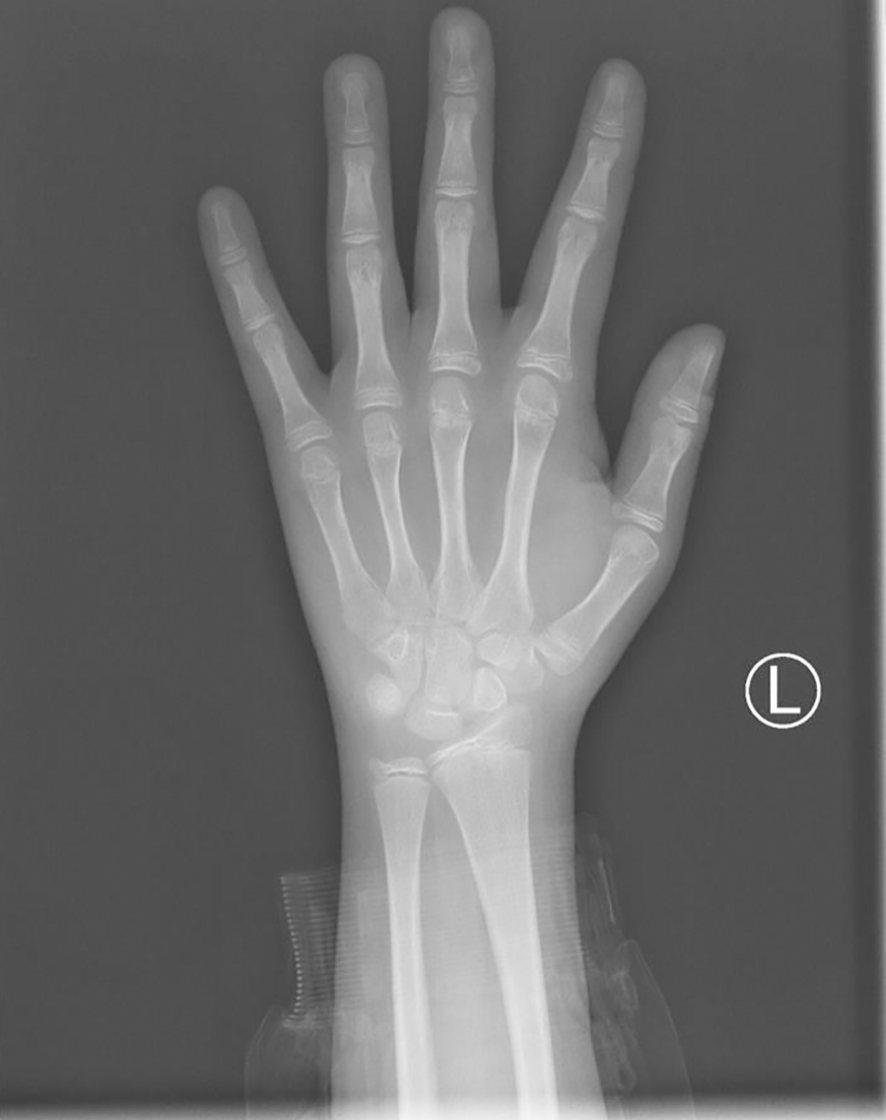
The bone age radiograph of the patient’s left wrist.
